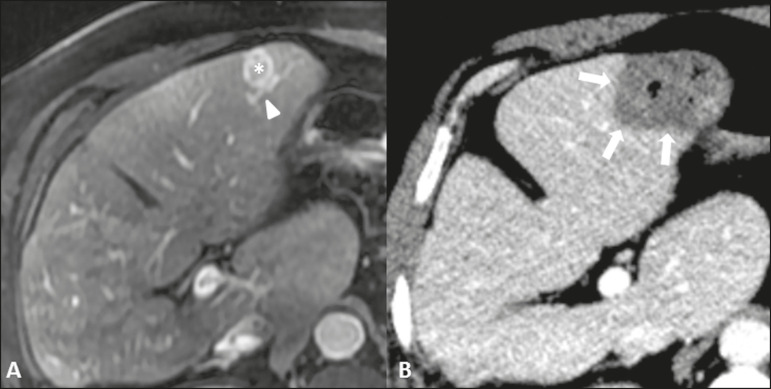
A: Magnetic resonance imaging scan of the upper abdomen, in the arterial phase, showing a lesion in liver segment III (asterisk). An arterial branch can be seen near the nodule (arrowhead). B: Follow-up computed tomography scan acquired after microwave ablation, showing a satisfactory ablation zone (arrows), with no heat-sink effect by the adjacent arterial branch (included in the ablation zone).
